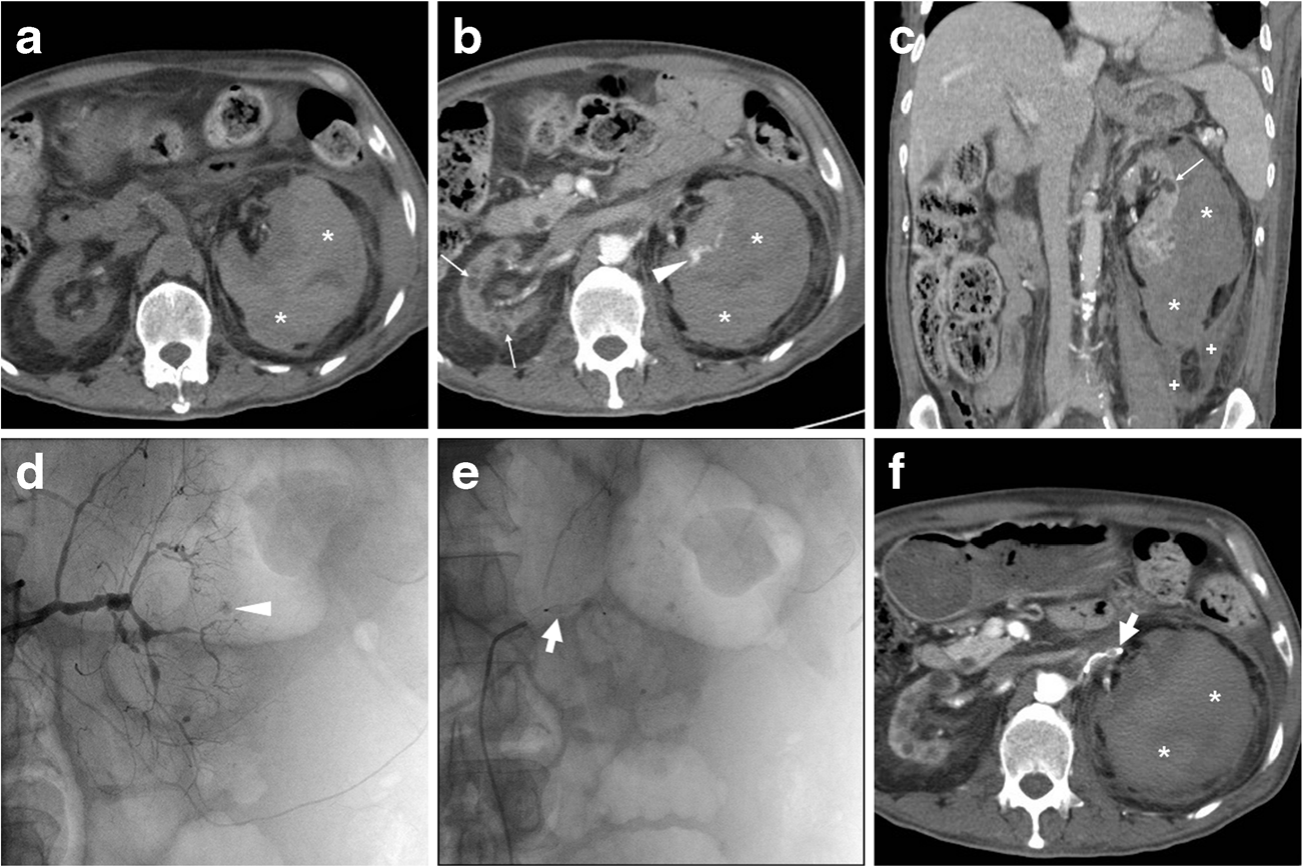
A 56-year-old Chinese man with hypertension, diabetes and chronic kidney failure undergoing regular haemodialysis suffered from acute abdomen pain with hypotension. Unenhanced (a), corticomedullary (b) and nephrographic (c) multidetector CT acquisitions showed a large left-sided subcapsular renal haematoma (*) and typical features of ESRD, including small cortical cysts (*thin arrows*). Note the minimal associated perirenal and posterior pararenal bloody effusion (+ in c). Focal CM extravasation consistent with active bleeding was detected by CT (*arrowhead* in b) and confirmed at selective renal angiography (*arrowhead* in d), originating from a distal arterial branch at the lower half of the kidney. Angiography revealed severe luminal irregularities of all renal arteries and allowed occlusion of the left renal artery with an 8-mm Amplatzer vascular plug (AGA Medical Corp., Plymouth, MN: *short arrows* in e) distally to the inferior adrenal artery. Follow-up CT (f) confirmed the Amplatzer plug in site (*short arrow*) and stopped haemorrhage
